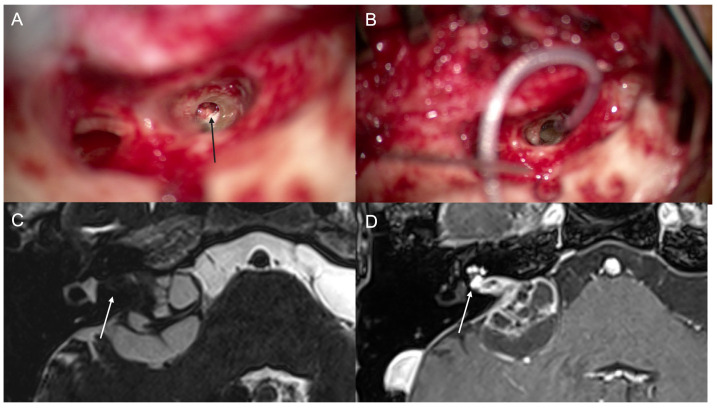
Case #4 (A–D). During the revision surgery, soft tissue was found in cochlea (black arrow) (A). After removal of the tissue, electrode was inserted (B). Postoperative T2-weighted MR with magnet removal 2 years later (C) shows a mass in IAC (white arrow) and CPA. T1-weighted MR with gadolinium enhancement shows enhanced mass in IAC and cochlea (white arrow) (D).
